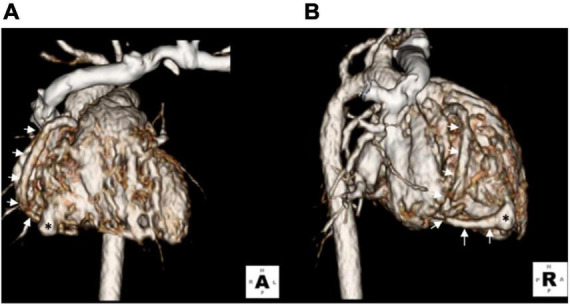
Three-dimensional computed tomography showing a lageniform coronary artery fistula (asterisks) arising from the RCA (arrows) drained into the inferior wall of the RV. (A) Anterior-posterior view. (B) Right lateral view.
